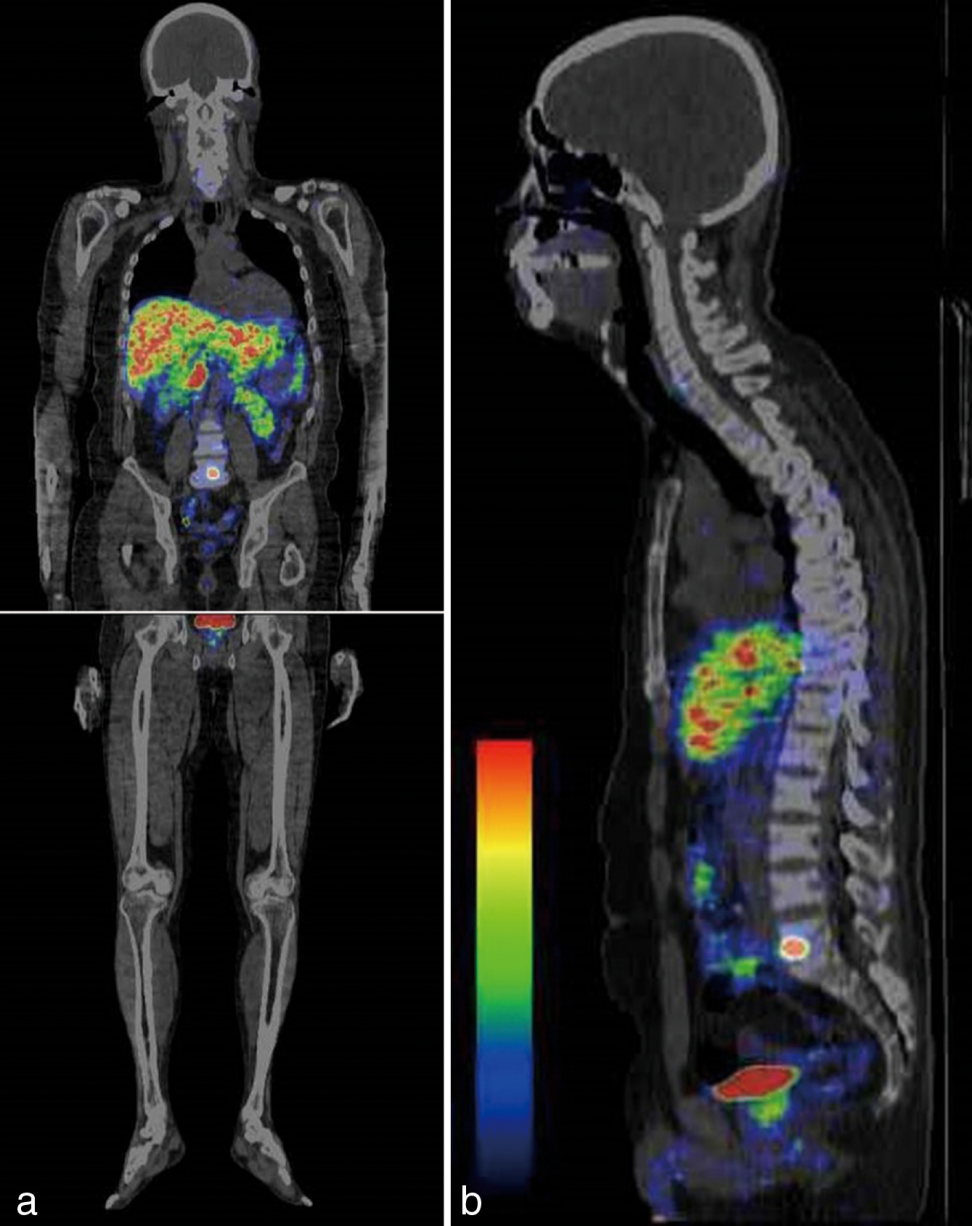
^68^Ga-DOTATOC positron emission tomography/CT scan (a, b) of the L5 vertebra demonstrates intense focal uptake within the tumour (maximum standardized uptake value = 10.5). ^68^Ga-DOTATOC positron emission tomography/CT scan did not show any abnormality in other regions.
